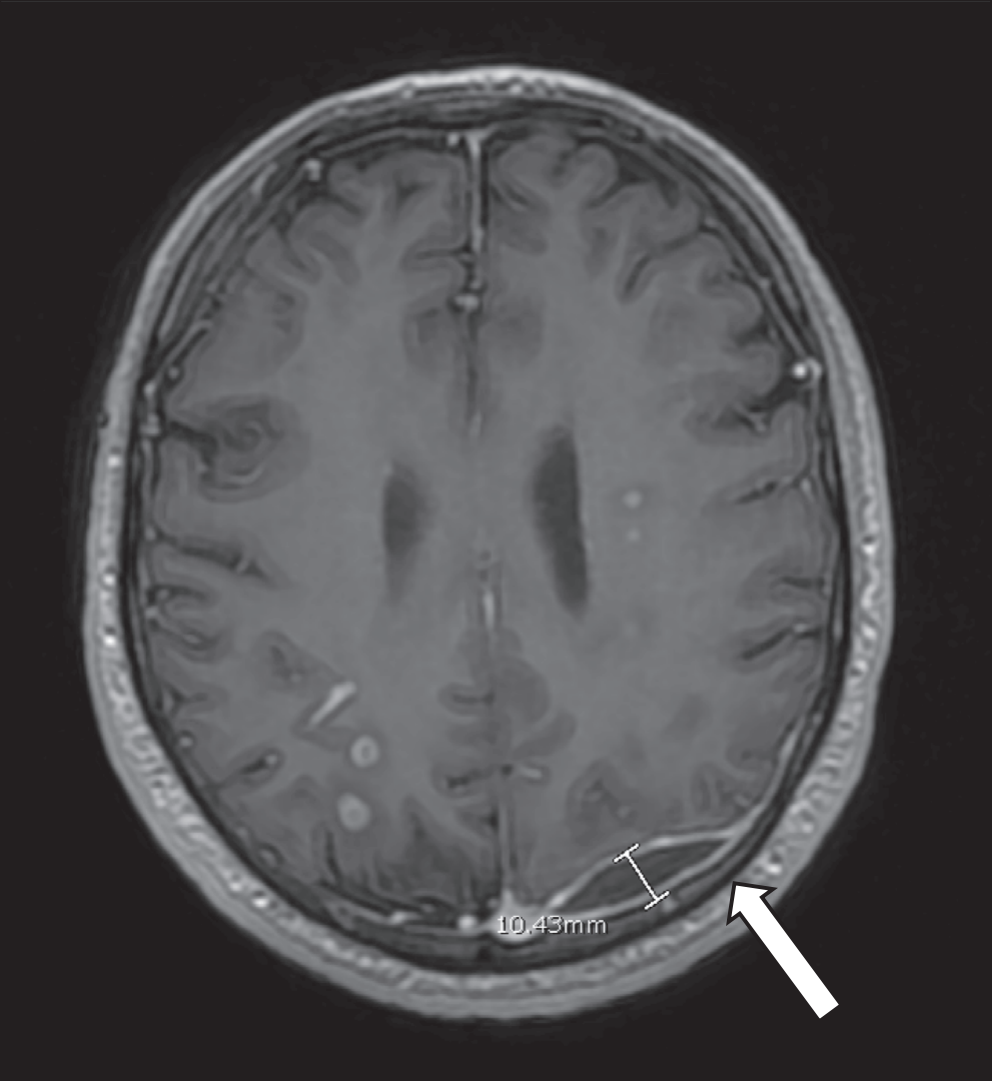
MRI Brain with increase in size of left occipital fluid collection to 11mm, prior to left occipital craniotomy
